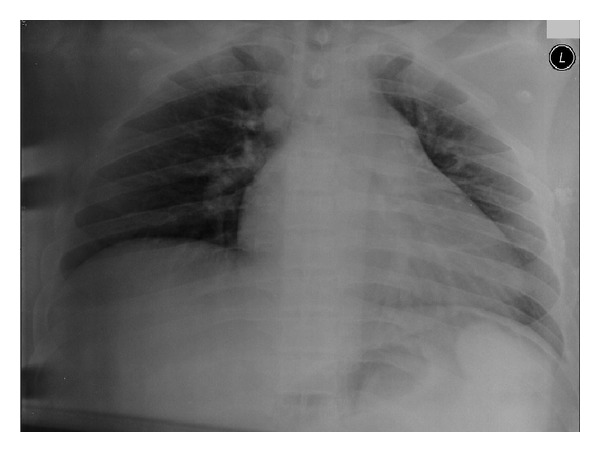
The initial chest X-ray on presentation to the emergency department shows no pathology.
